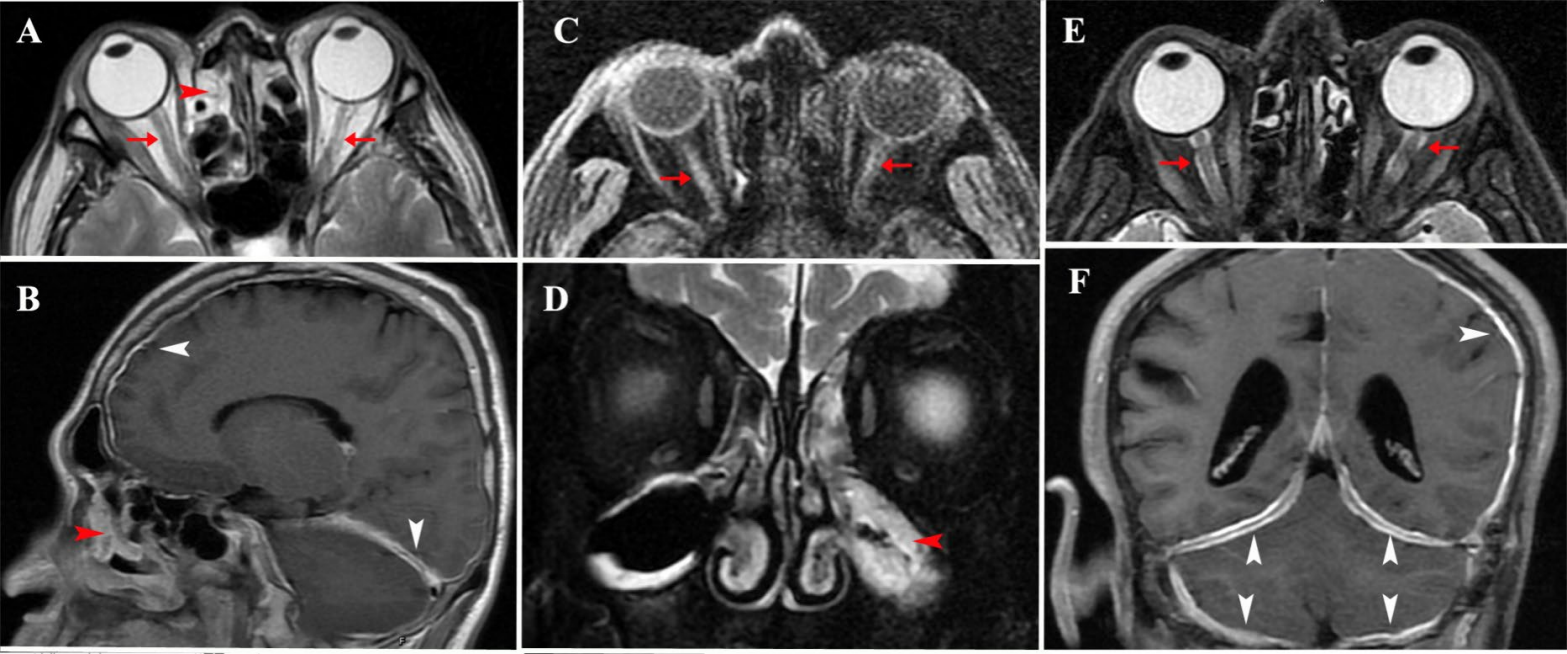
A, B from a 49-year-old man. A, axial T2-weighted MRI show hyperintense signals at the bilateral optic nerve (red arrow) and right ethmoid sinus (red arrowhead). B: Sagittal T1-weighted postcontrast MRI shows enhancement signals of the maxillary sinus (red arrowhead) and dura mater (white arrowhead). C, D from a 71-year-old man. C, axial T1-weighted postcontrast MRI shows enlargement and enhancement of bilateral optic nerves (red arrow). D Coronal T2-weighted MRI shows a hyperintense signal at the left maxillary sinus (red arrowhead). E, F from a 58-year-old man. E: Axial T2-weighted MRI shows hyperintense signals at bilateral optic nerve and sneath (red arrow). F, Coronal T1-weighted postcontrast MRI show the dura mater of bilateral frontal lobes, bilateral tentorium cerebelli parietal, the left parietal, and the left temporal lobes were thickened and enhanced (white arrowhead)
